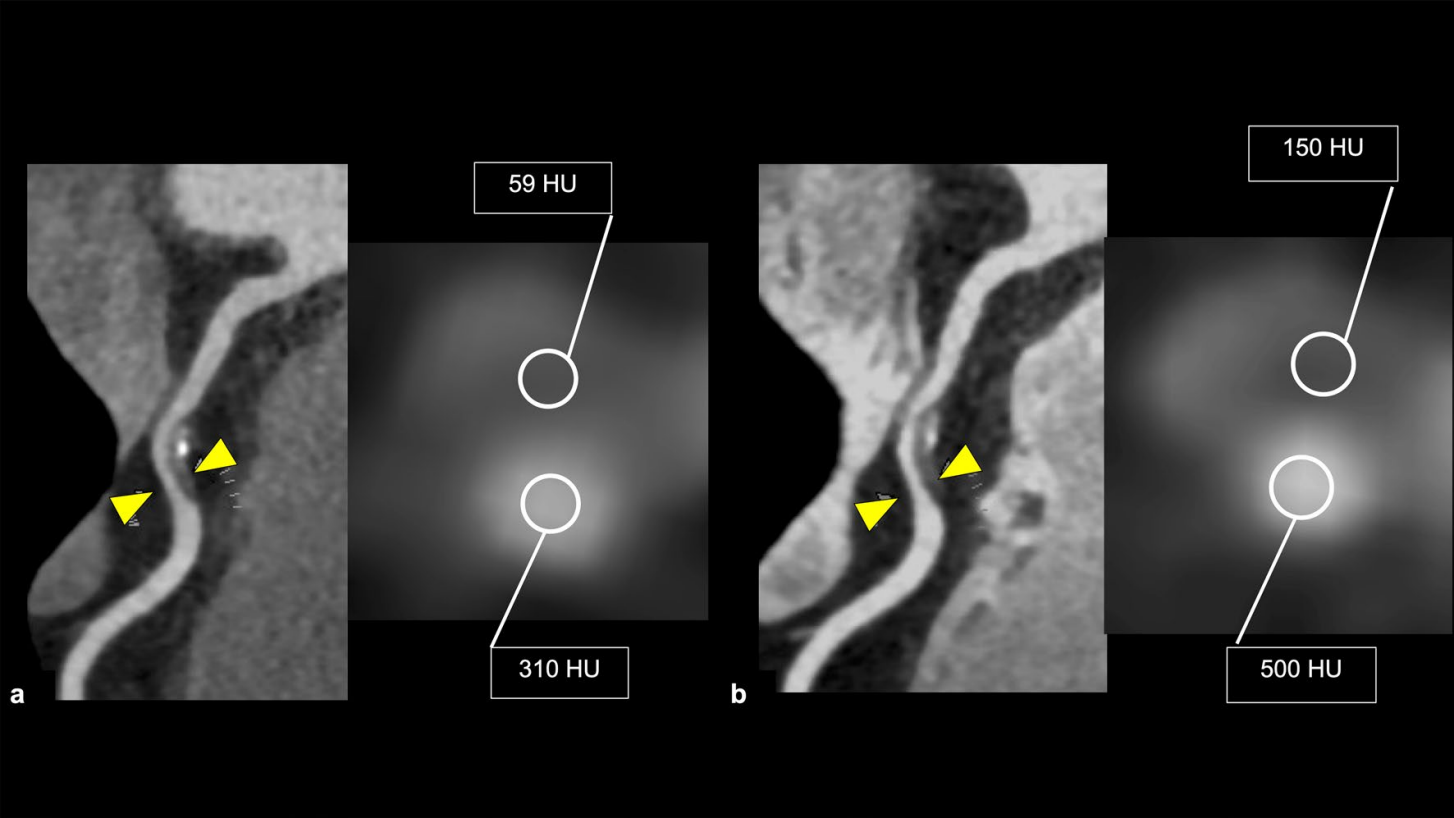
Changes in coronary plaque CT attenuation due to CCTA scanning condition. Two CCTAs are performed in a short interval with the same scan parameters (a: first scan, b: second scan). CT attenuation of coronary plaque differs between the first and second scans due to the difference in the CT attenuation of coronary artery lumens. *CT* computed tomography, *CCTA* coronary computed tomography angiography
